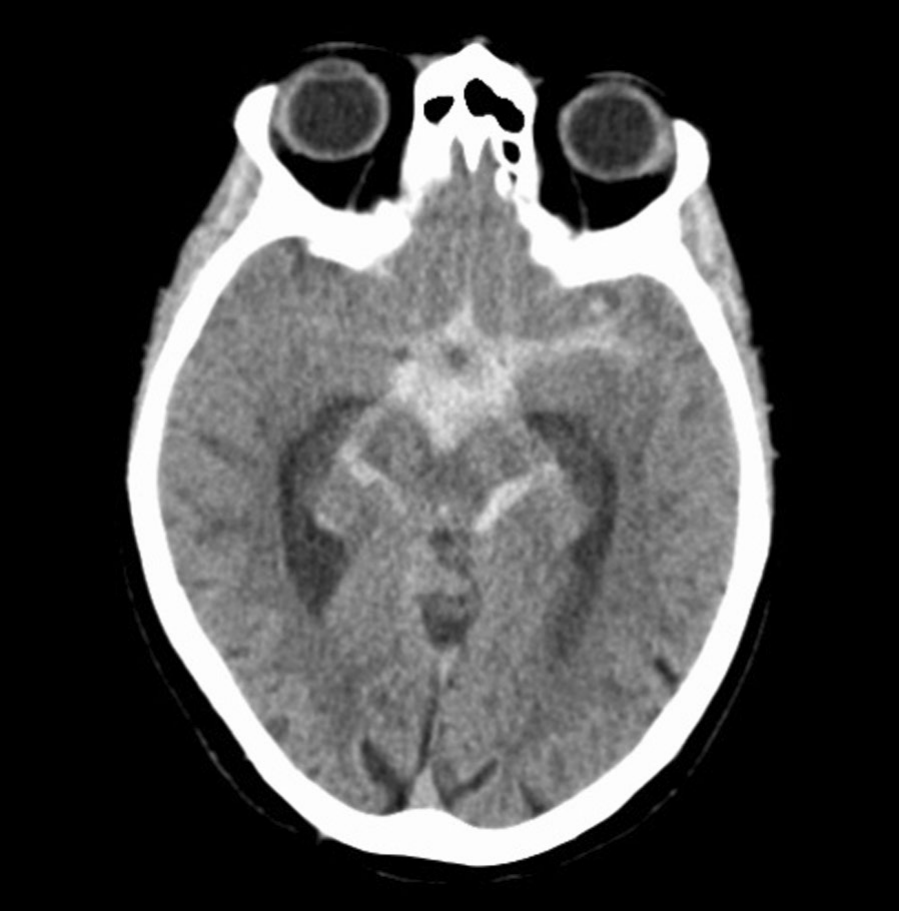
Axial head computed tomography showing subarachnoid hemorrhage with thick blood filling the basal cisterns
